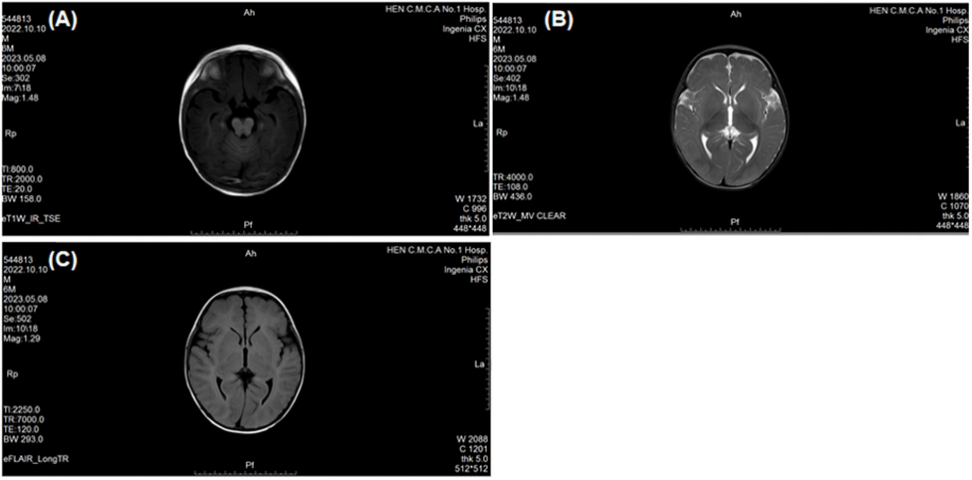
Brain MRI (3.0T) reveal: (A) Linear hypointense signal in the right frontal lobe (likely vascular shadow); (B) Bilateral frontotemporal subarachnoid space widening; (C) Bilateral otomastoiditis.
